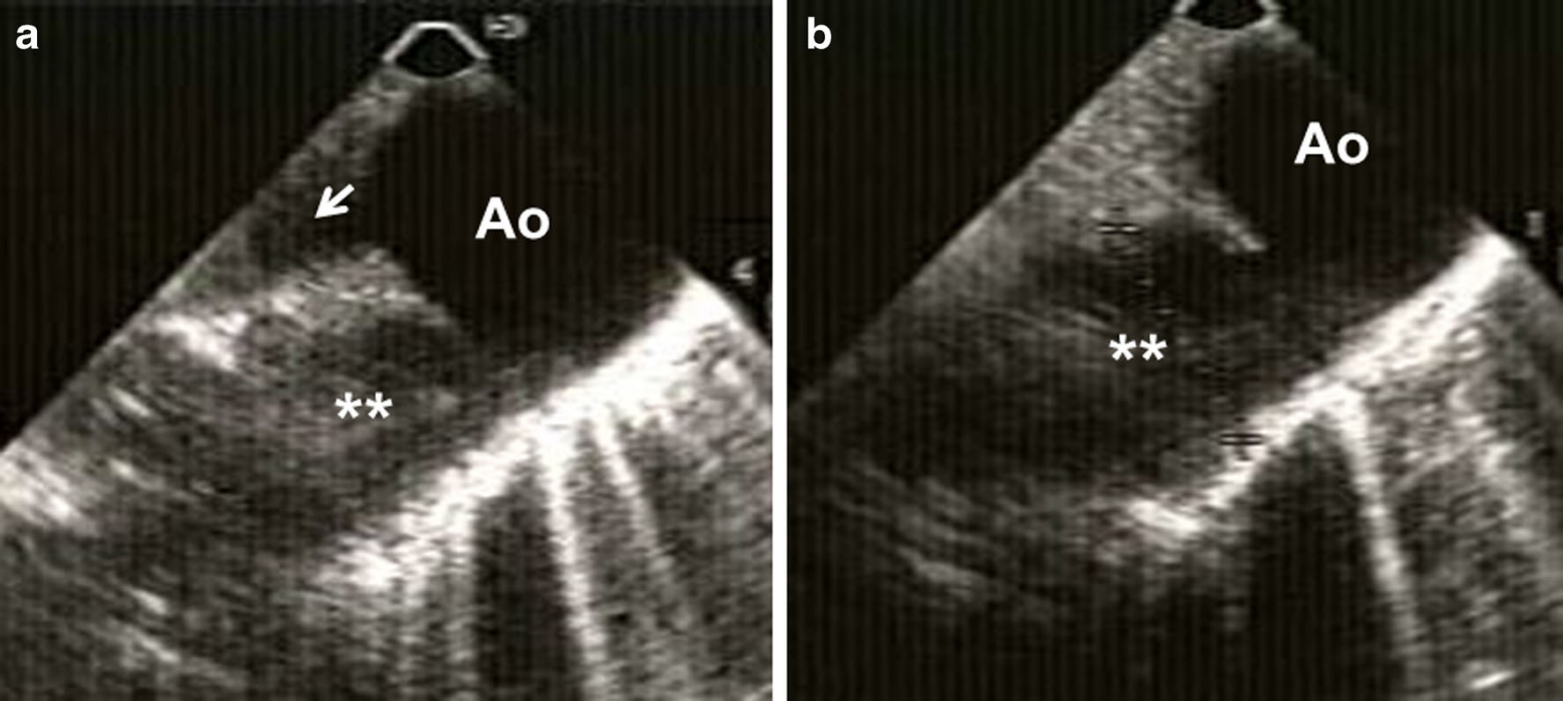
Due to fetal distress and maternal hypoxemia, a semi-emergent cesarean section was performed and a 28-week live female fetus was delivered. Intraoperative transesophageal echocardiographic images showing a large (1.8 cm in diameter) patent ductus arteriosus (*double white asterisks*) connecting with proximal thoracic aorta (*Ao*) (a). b shows aortic arch (*white arrowhead*). There was bidirectional flow across PDA. On postoperative day 2, patient underwent re-exploration of abdomen due to marked hypotension and marked cyanosis (reversal of shunt from PDA to aorta due to hypotension), and large amounts of clot were removed from the abdomen. Subsequently, the patient developed thrombocytopenia, disseminated intravascular coagulation, and elevated liver enzymes and died within 24 h
